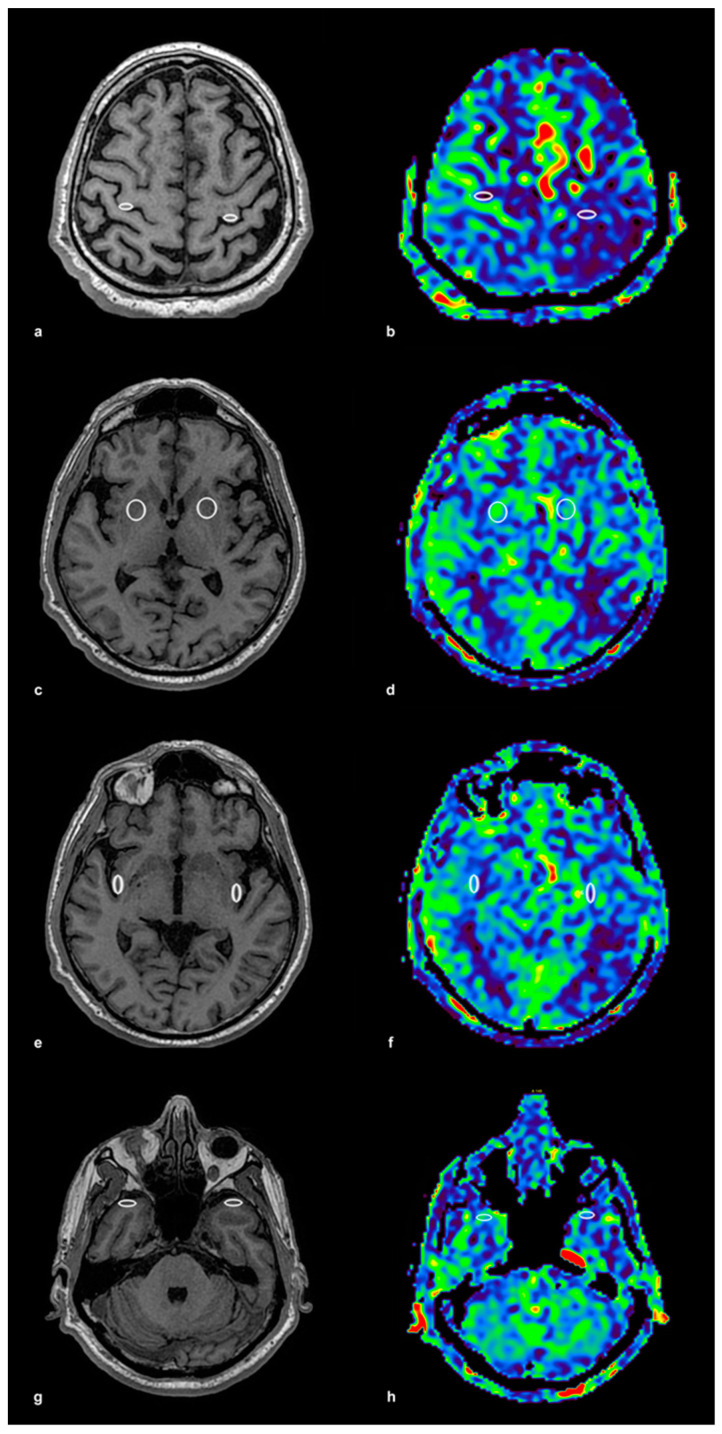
ROI Placement and ASL Perfusion Map in a Patient with Carotid Stenosis. This figure presents ROI placement and ASL perfusion maps in a patient with unilateral carotid stenosis, following the same methodology as in Figure 1. (a) T1-weighted image with ROI placement in the precentral gyrus (frontal cortex) bilaterally, and (b) the corresponding ASL perfusion map with the same ROIs transferred for CBF quantification. (c) T1-weighted image with ROI placement in the lentiform nucleus bilaterally, and (d) the corresponding ASL perfusion map. (e) T1-weighted image with ROI placement in the insular cortex bilaterally, and (f) the corresponding ASL perfusion map. (g) T1-weighted image with ROI placement in the temporal cortex bilaterally, and (h) the corresponding ASL perfusion map. ROI sizes (15–40 mm^2^) were adjusted according to the anatomical region, with elliptical ROIs applied in thinner cortical regions and circular ROIs in larger areas. The colored ASL perfusion maps (b,d,f,h) represent quantitatively measured cerebral blood flow (CBF), with warmer colors (red, yellow) corresponding to higher CBF values and cooler colors (green, blue) indicating lower values.
